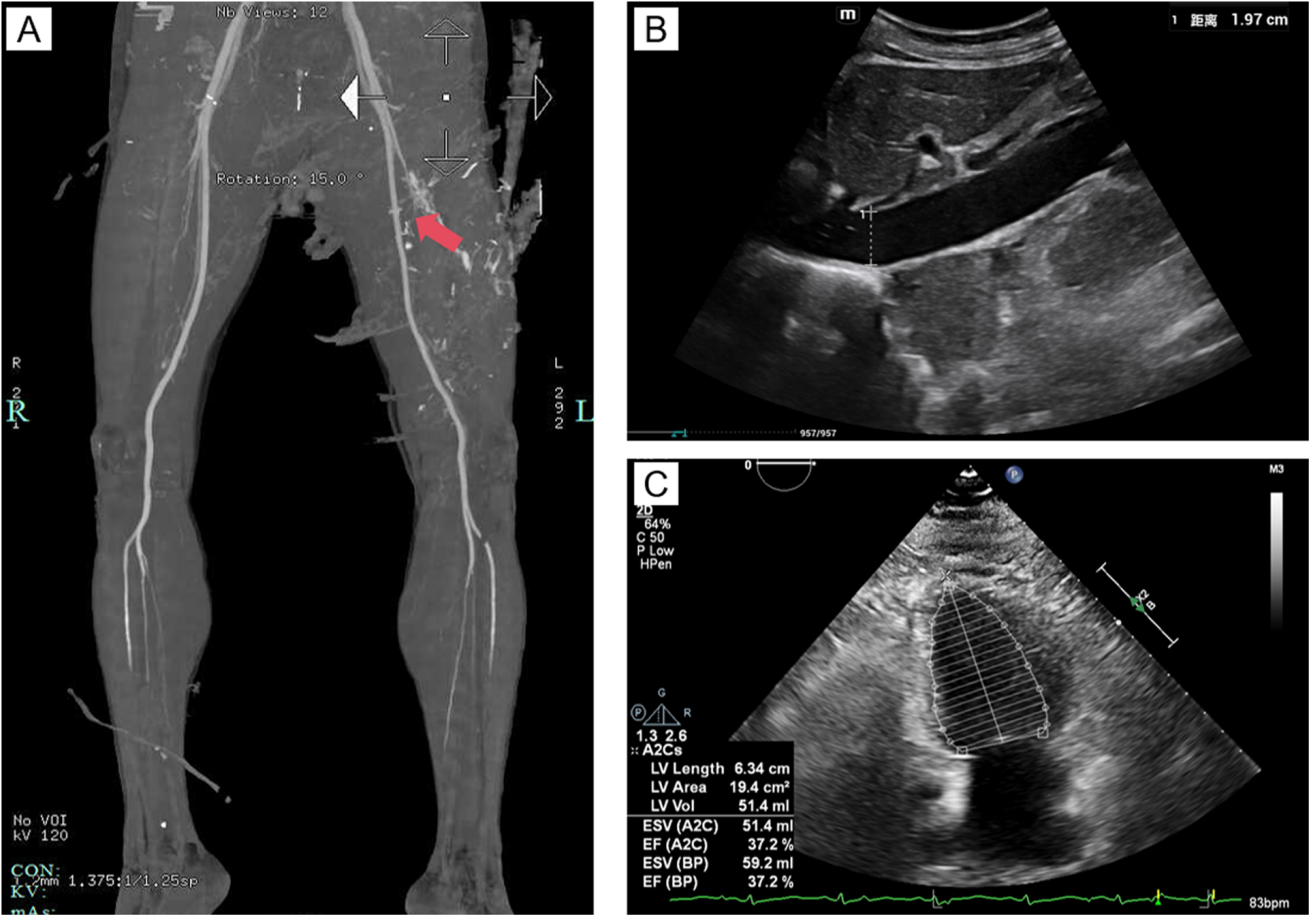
Imaging findings. (A) Contrast-enhanced CT of the lower extremities, with the red arrow indicating rupture and bleeding of the left femoral artery, with extravasation of contrast agent visible; (B) Ultrasound image of the inferior vena cava; (C) Echocardiographic image of the heart.
